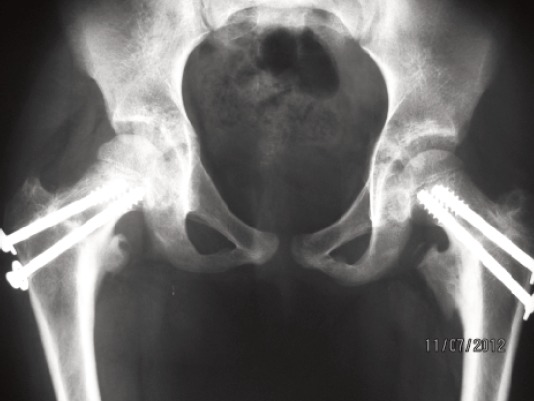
Anterioposterior radiograph of pelvis showing both
